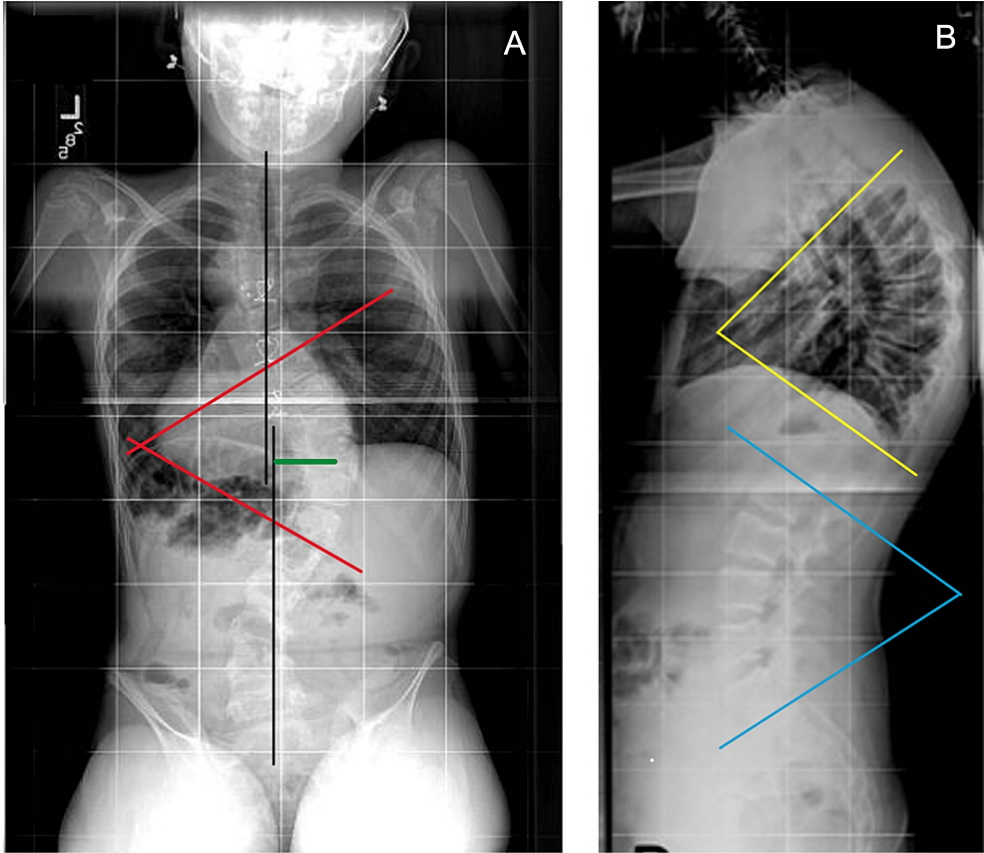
Representative radiographs with measurements. A: Red angle - coronal Cobb angle; upper black line - C7 plumb line; lower black line - central sacral line; green line - apical vertebral translation; distance between black lines - global coronal balance. B: Yellow angle - thoracic kyphosis; blue angle - lumbar lordosis.
